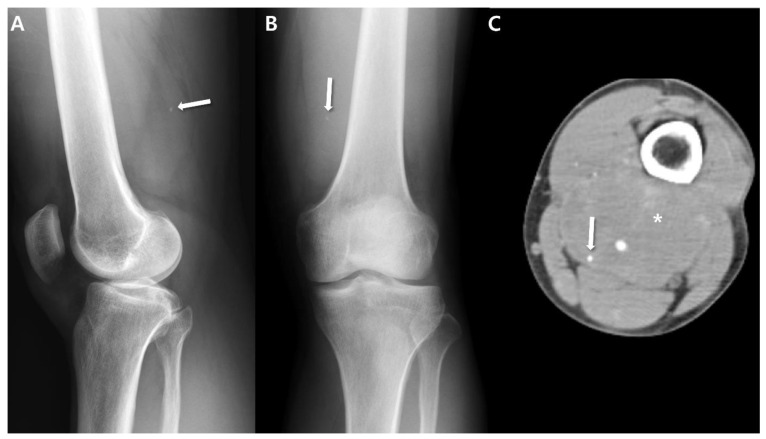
Stippled calcification in synovial sarcoma of 28-year-old man with synovial sarcoma (monophasic type): (A) Lateral radiograph of knee shows stippled calcification (arrow) in soft tissue opacity of distal femur. (B) Anteroposterior radiograph also shows stippled calcification (arrow) in soft tissue opacity. (C) Lower extremity CT angiography shows stippled calcification (arrow) in heterogenous enhancing mass (*) of distal femur.
